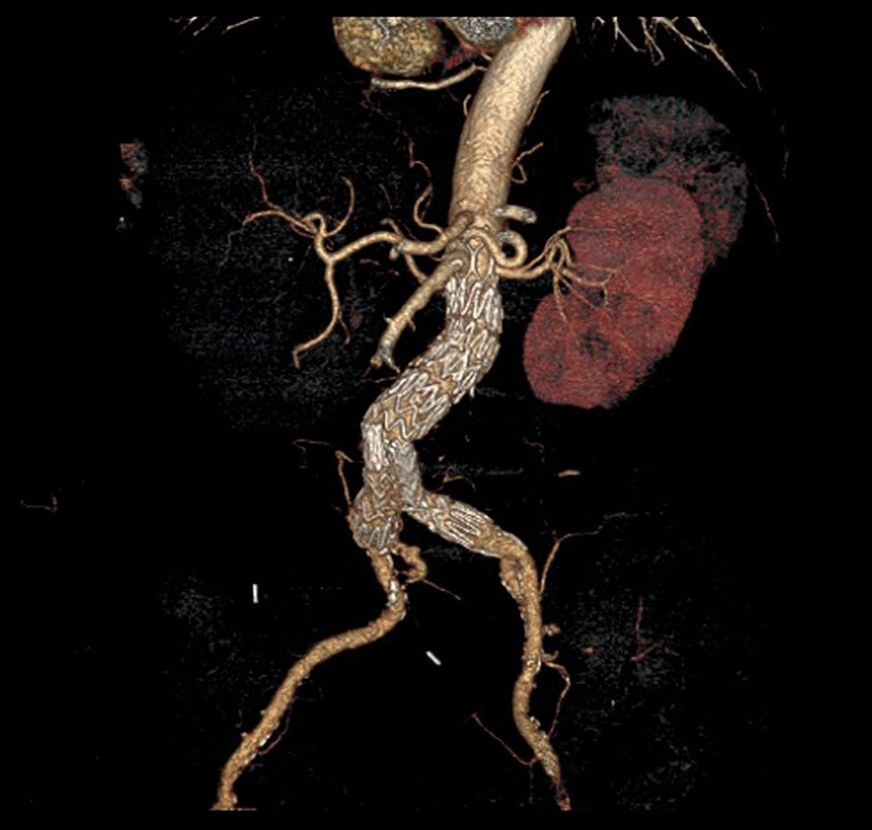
Postoperative CT-scan after redo EVAR
